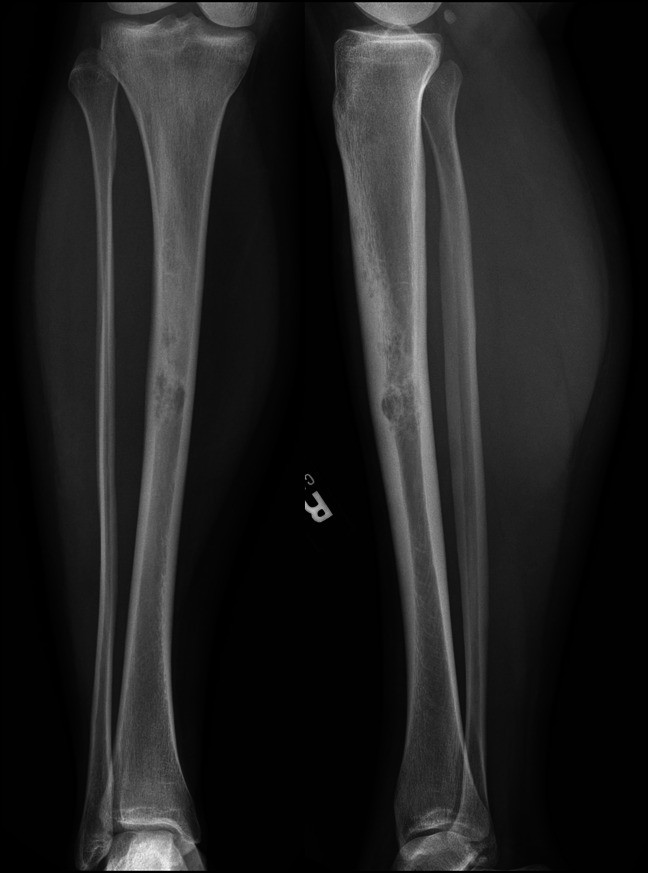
Radiograph of 28-year-old woman demonstrating poorly defined, mixed, cortically based lesion with endosteal scalloping without apparent cortical destruction (case 2: AP and lateral radiographs of diaphyseal tibia lesion).
